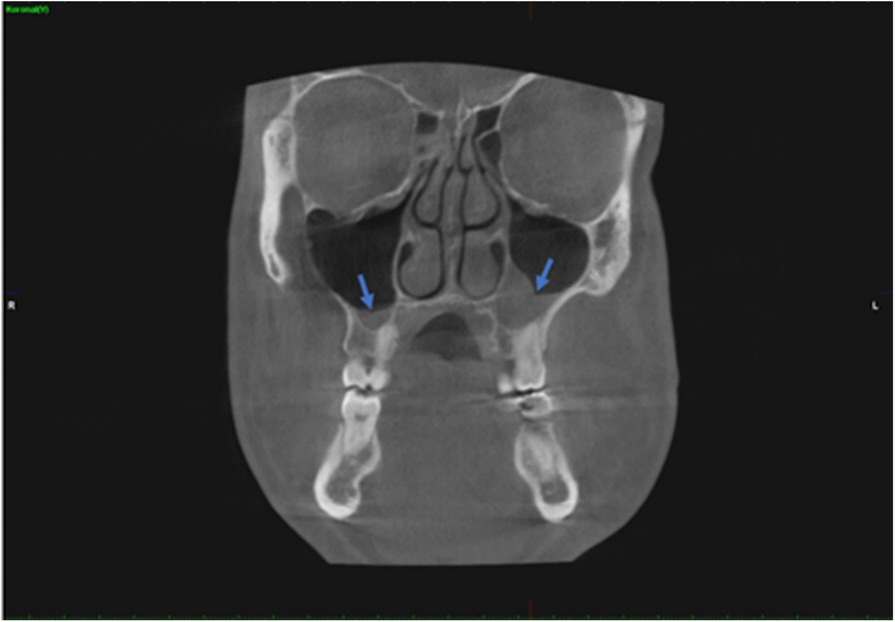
Determination of the presence of maxillary sinus pathology in the coronal CBCT images
